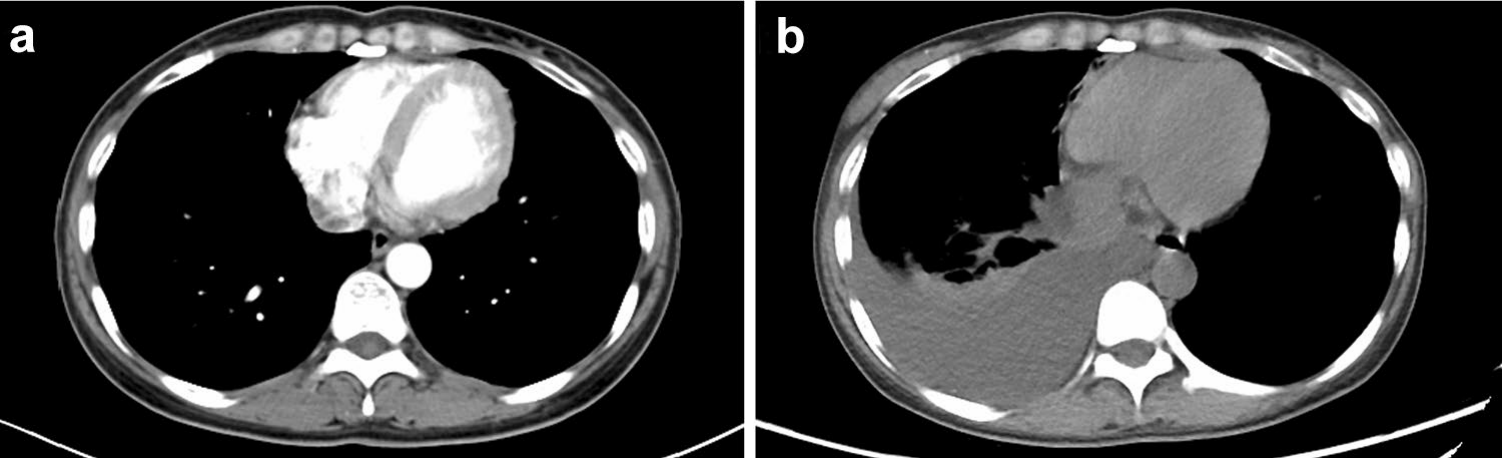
Computed tomography scan in ceritinib withdrawal (a), and disease progression in September 2019 in patient 2 (b)
